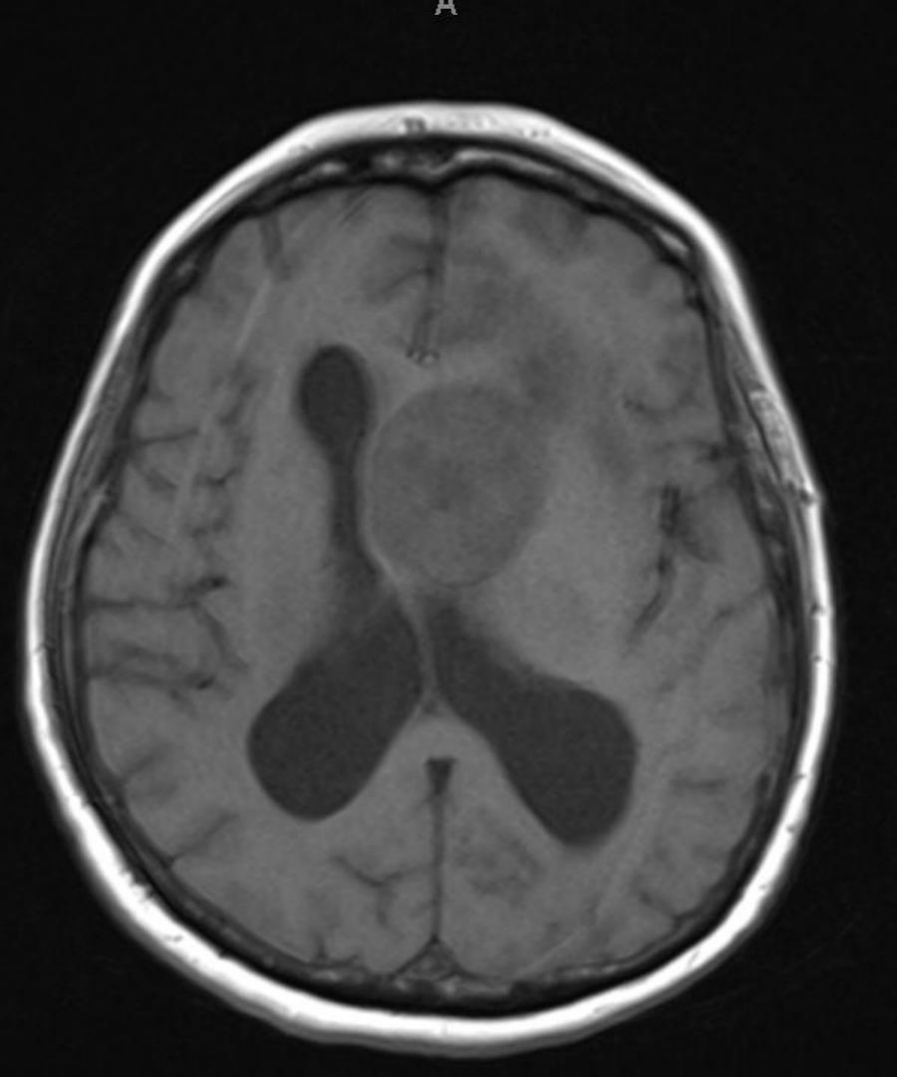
Initial MRI (pre-contrast) T1 axial pre-contrast MRI showing a hypodense lesion in the left lateral ventricle.
